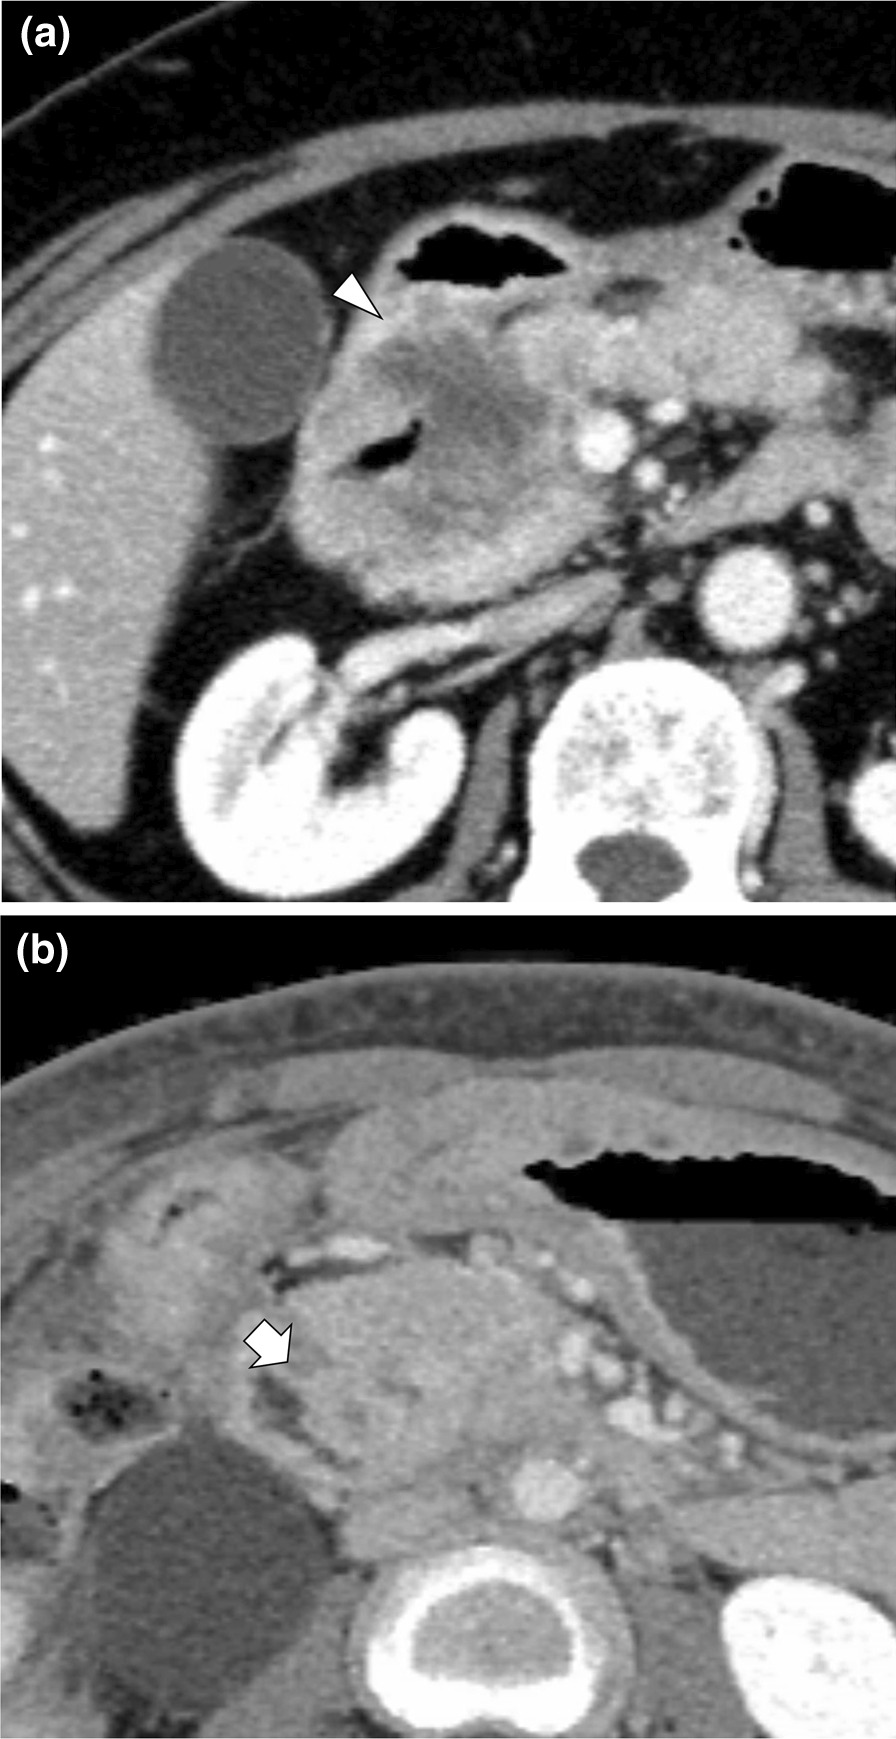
Computed tomography images showing tumor invasion to the main duodenal papilla a Pancreatic cancer is shown invading the main duodenal papilla directly (arrowhead). b Duodenal infiltration of the uterine cancer is shown invading the main duodenal papilla (arrow)
